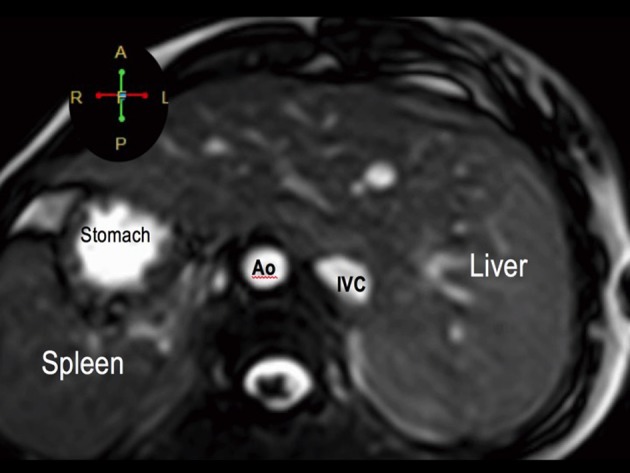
Visceral situs inversus seen on cardiac MRI. Axial cut at the level of the left-sided liver and right-sided spleen and stomach. The aorta (Ao) is on the right while the inferior vena cava (IVC) is on the left.
